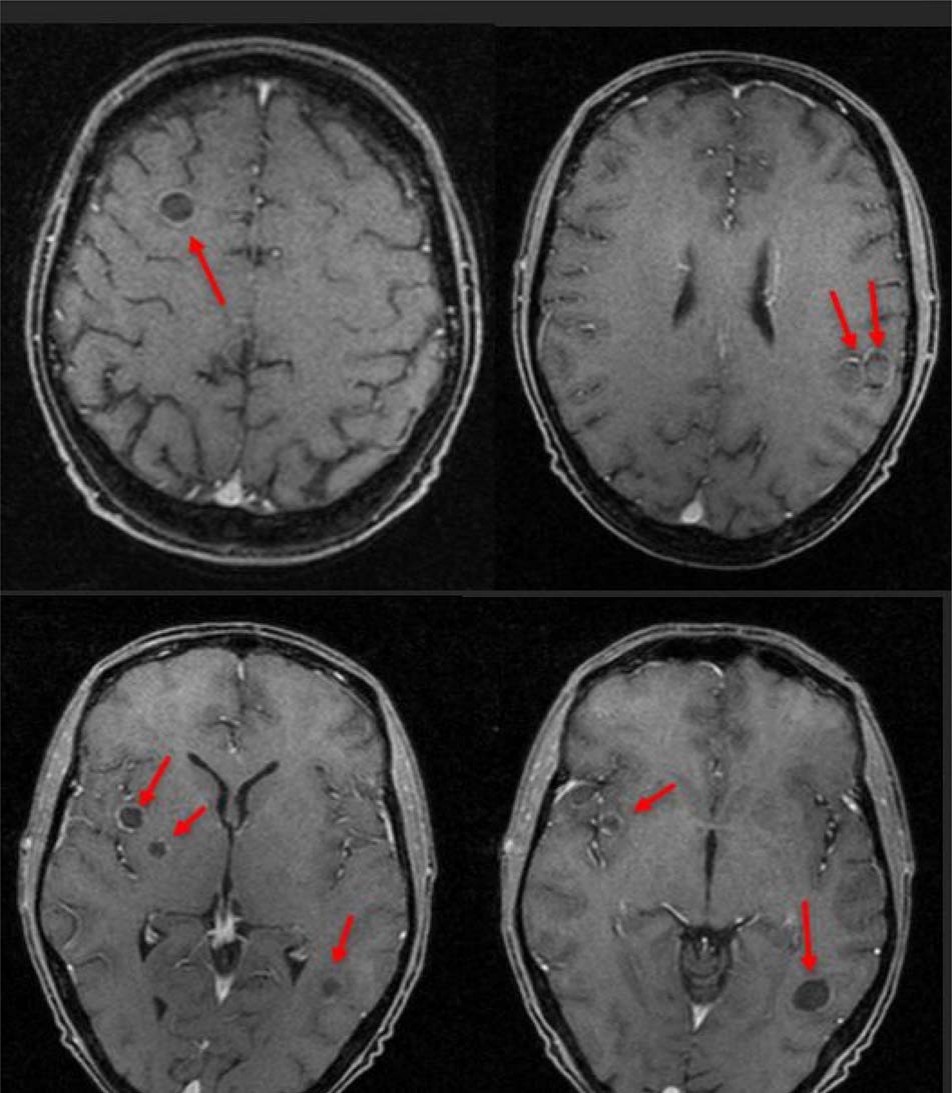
MRI scan after first seizure showing multiple brain abscesses.
